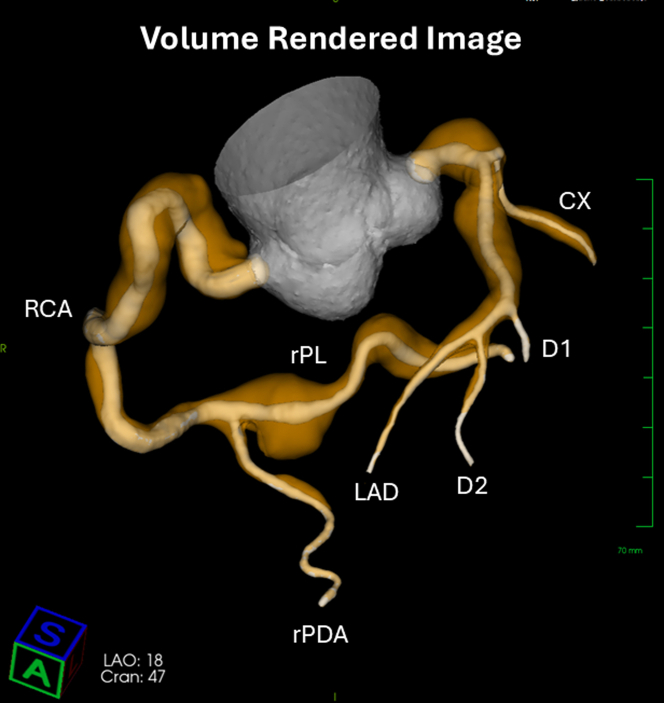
Volume-Rendered CCTA Image Reconstruction Demonstrating Diffuse, Triple-Vessel Coronary Artery Disease CCTA = coronary computed tomography angiography; CX = circumflex artery; D1 = first diagonal branch; D2 = second diagonal branch; LAD = left anterior descending artery; RCA = right coronary artery; rPDA = right posterior descending artery; rPL = right posterolateral branch.
